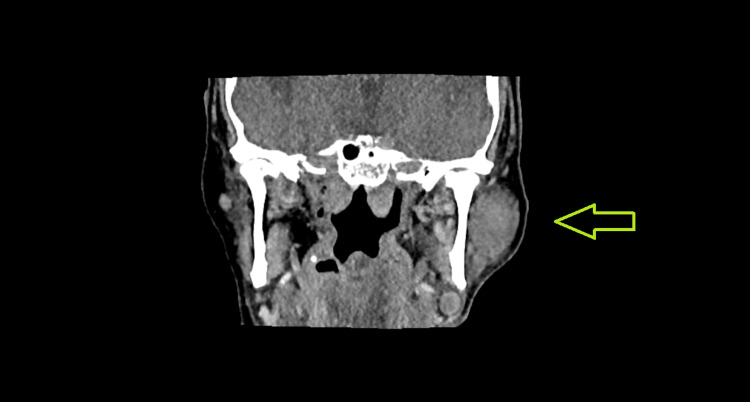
CT showing myeloid sarcoma in the parotid gland.
